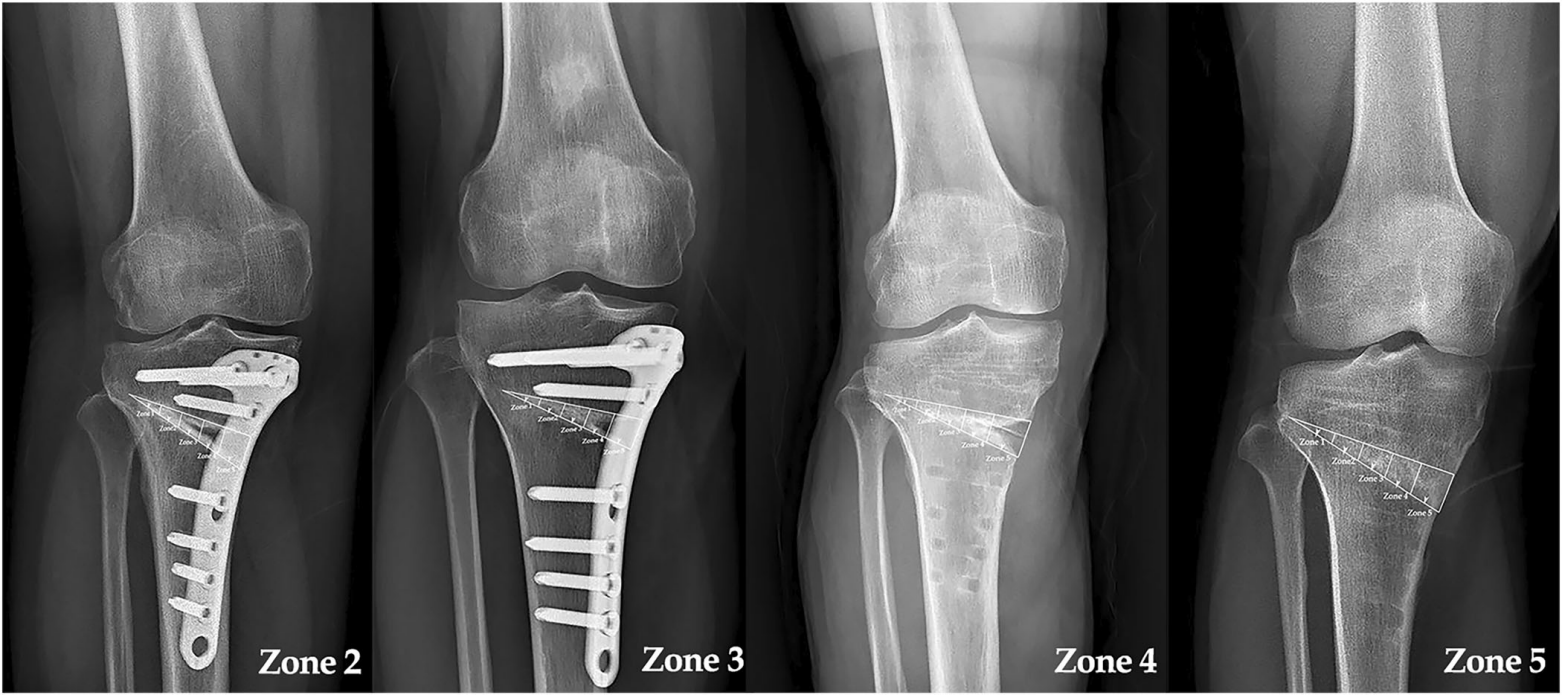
Different bone union zones at 1‐year follow‐up. Anteroposterior radiographs showing different bone union progressions from zone 2 to zone 5 at 1‐year follow‐up. From left to right, the gaps were filled with allograft, allograft, no graft, and autograft, respectively. When the gap was larger than 80%, filled hardware removal was considered.
